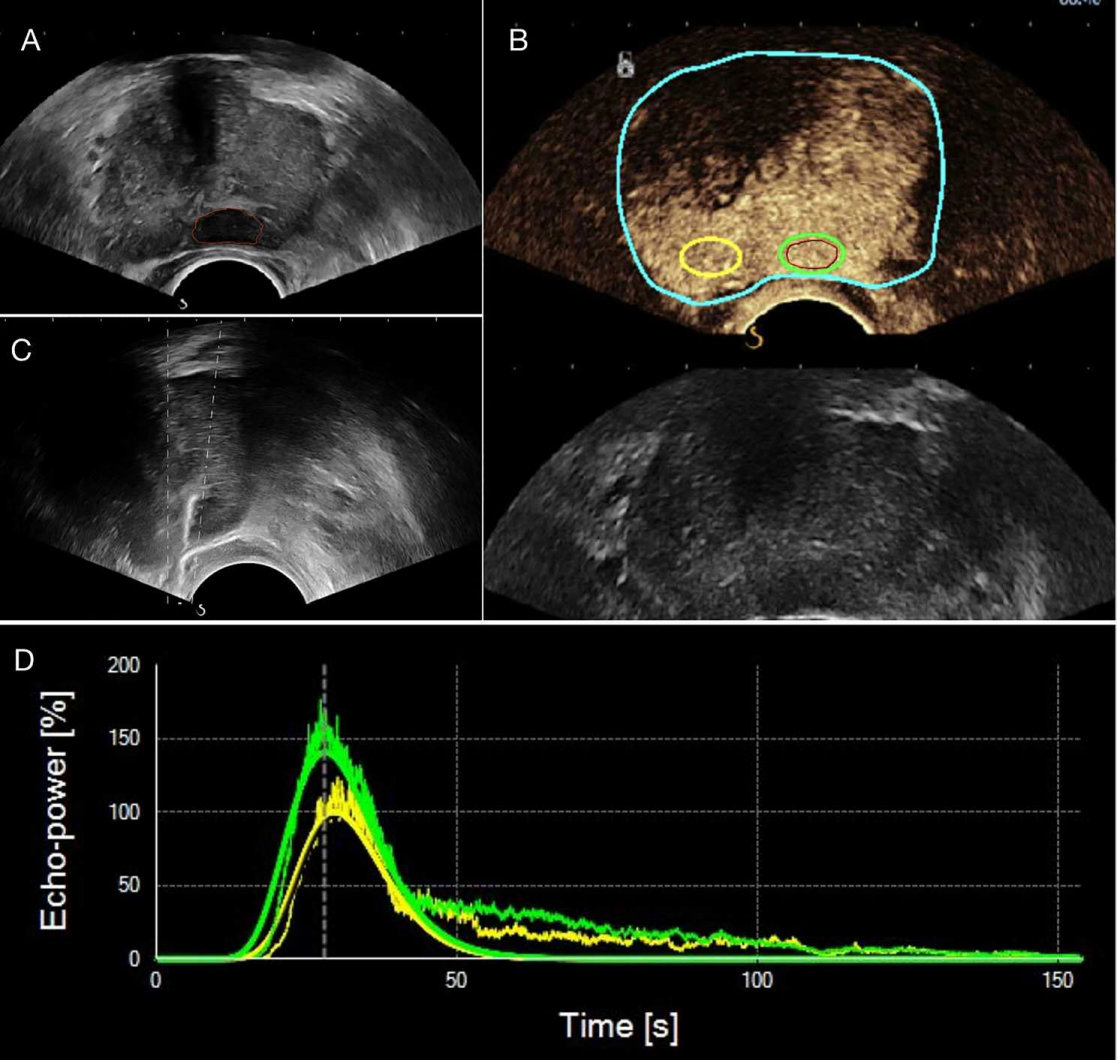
Example image of a 68-year-old PCa patient with an elevated PSA of 6.3 ng/mL and a hypoechoic lesion in the left PZ of the prostate. a B-mode, b CEUS mode, c target TRUS biopsy, and d the time-intensity curve. a and b represent ROI placement within the target lesion, which is depicted in the red circle. In b, the analysis and reference ROIs are encircled in green and yellow, respectively. *CEUS* contrast-enhanced ultrasound, *TRUS* transrectal ultrasound, *ROI* region of interest
